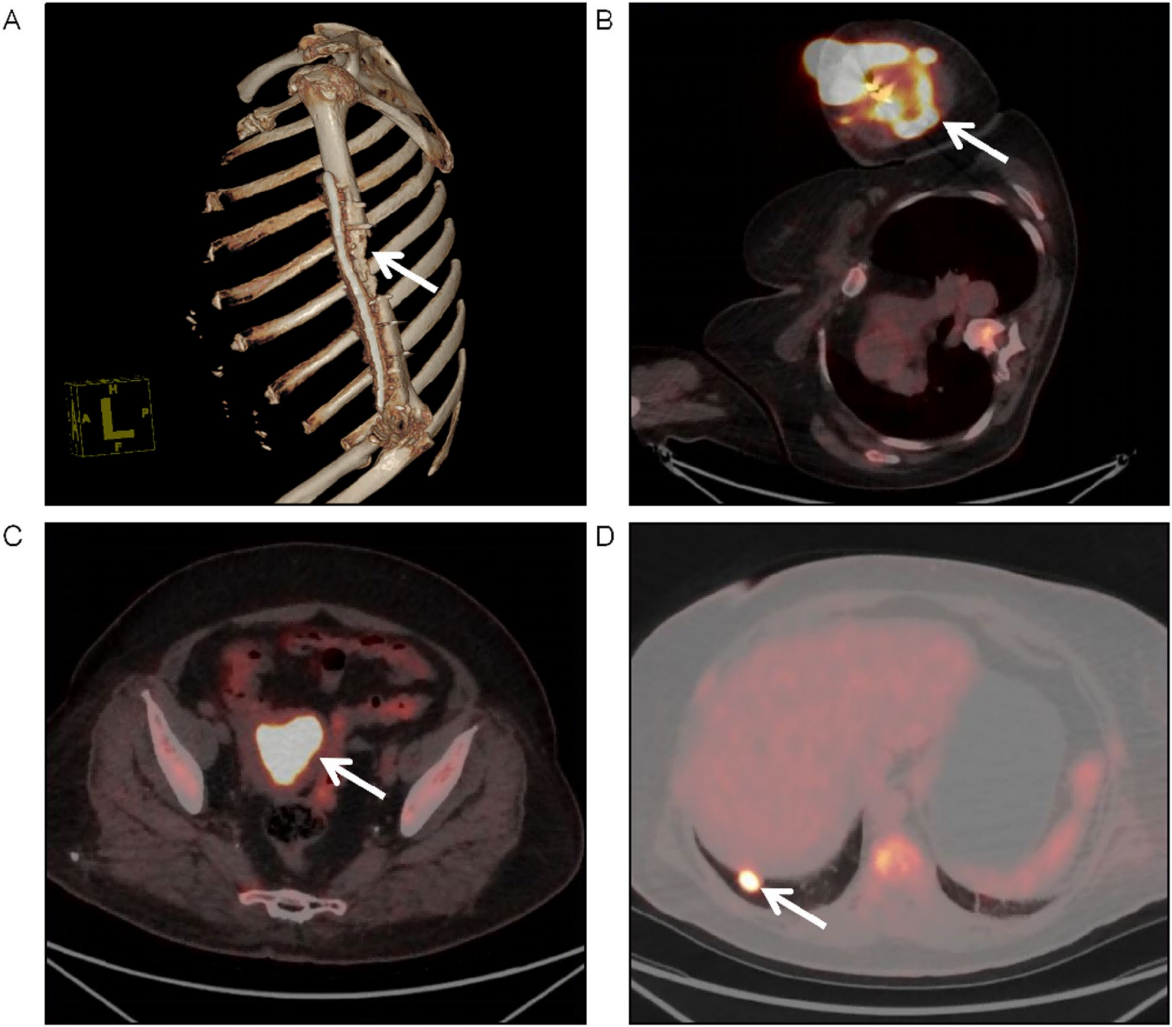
Radiographic evaluation before primary surgery. A Three-dimensional bone imaging of the left upper arm revealed a localized cortical defect in the left humerus, with an irregular bone contour in the humeral head. B-D Fused PET-CT images at presentation. B A large, hypermetabolic soft tissue mass (9.3 × 7.1 cm, SUVmax: 21.79) surrounding the left humeral shaft (arrow). C An intrauterine space-occupying lesion (4.5 × 3.4 cm, SUVmax: 14.31) with intense FDG uptake (arrow). D A hypermetabolic lung nodule (1.5 cm in diameter, SUVmax: 14.03) in the right lower lobe (arrow)
